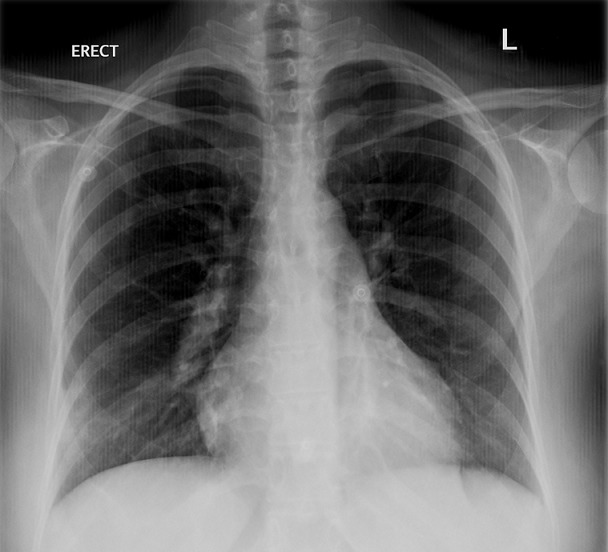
Chest X-ray PA view was reported normal
